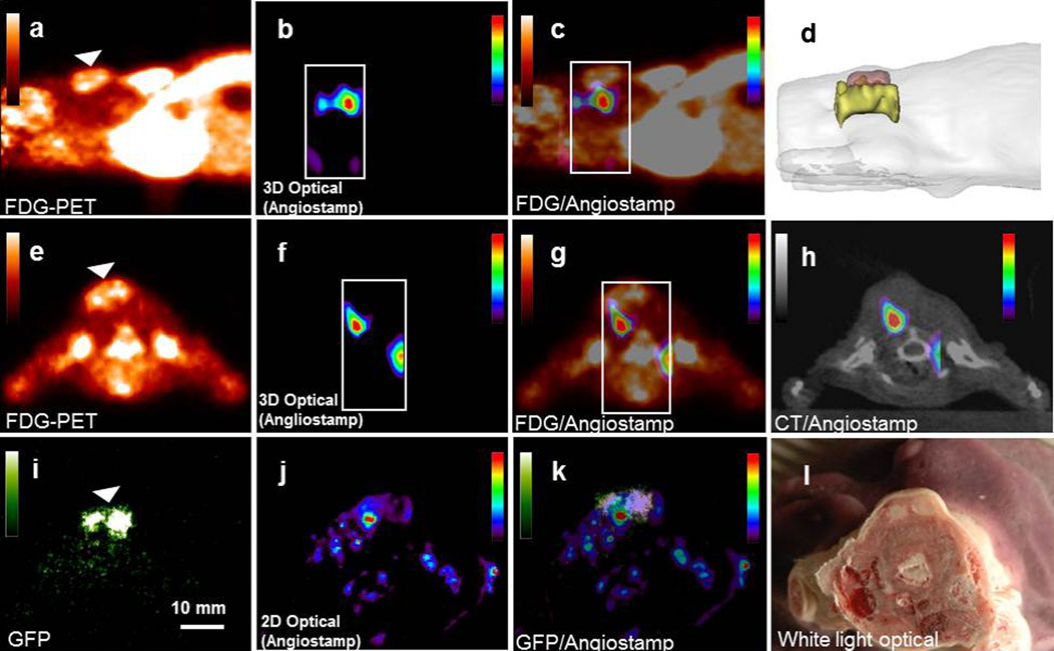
Imaging using Angiostamp and [^18^F]FDG. (a) Sagittal slice of [^18^F]FDG PET at the level of the tumor (with a tumor volume of 59 mm^3^). The *arrow* pinpoints the position of the tumor as identified by [^18^F]FDG. (b) Sagittal fDOT image of Angiostamp. The area, in which the fDOT reconstruction is performed, is depicted by a *dotted white*
